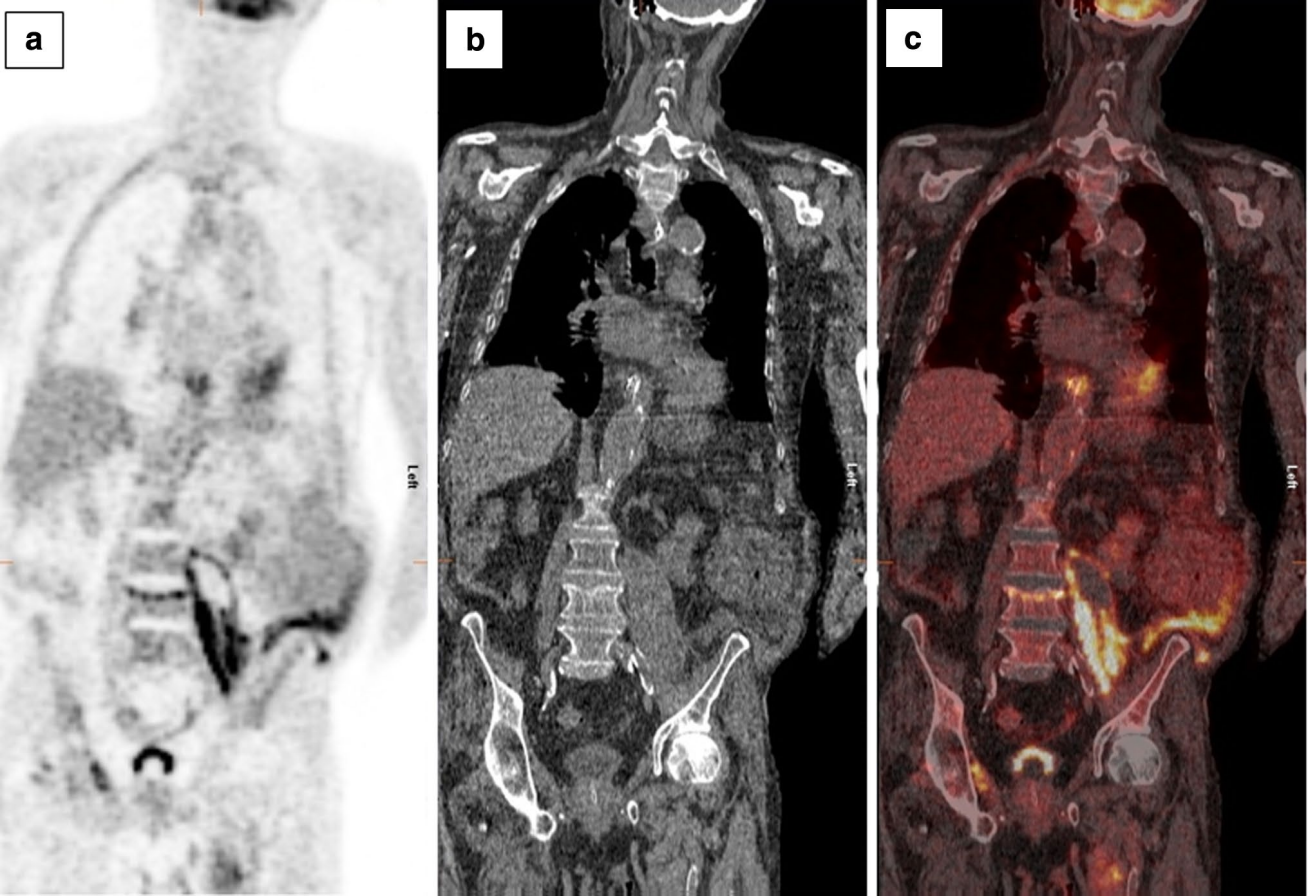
^18^F-fluorodeoxyglucose positron emission tomography (^18^FDG-PET) (a), low-dose CT (b), and integrated ^18^FDG-PET/CT (c) images, demonstrating increased FDG-uptake in the abscess formation in the left iliopsoas muscle, extending into the intervertebral space cranially of L4 and into the adipose tissue reaching the left abdominal wall. The ^18^FDG-PET could not be assessed for disseminated lesions in the brain due to a motion artifact of the head during the procedure
